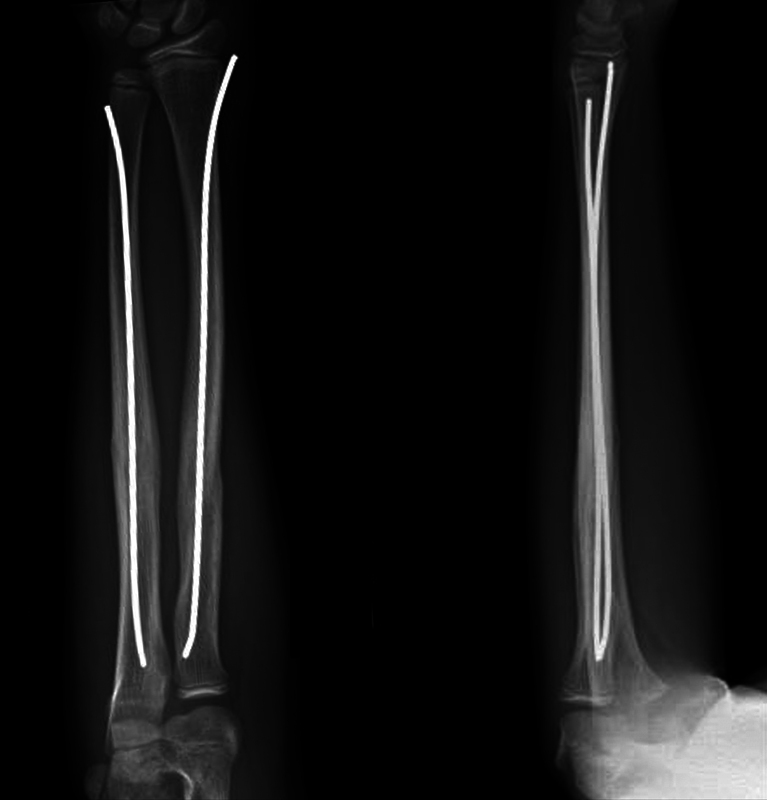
Anteroposterior and lateral radiograph at 6 months follow-up.
